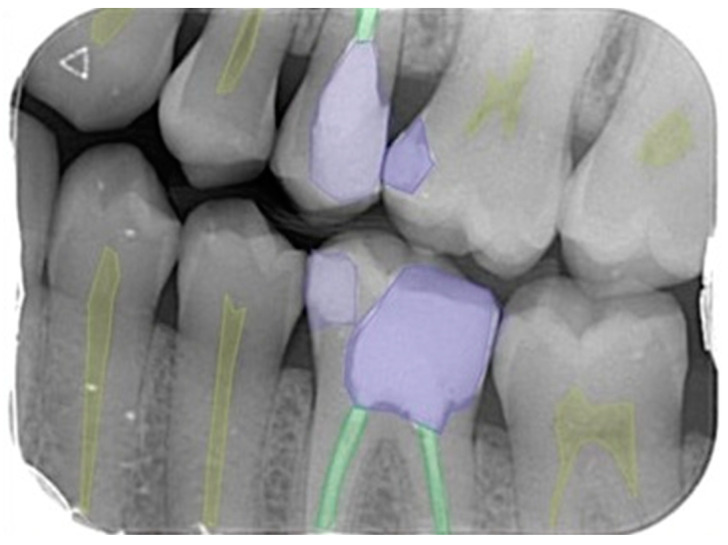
Polygonal style on bite-wing radiography image; labeling for 5 different dental diagnoses as dental pulp, dental caries, dental restorative filling material, dental crown, and dental root canal filling material.
